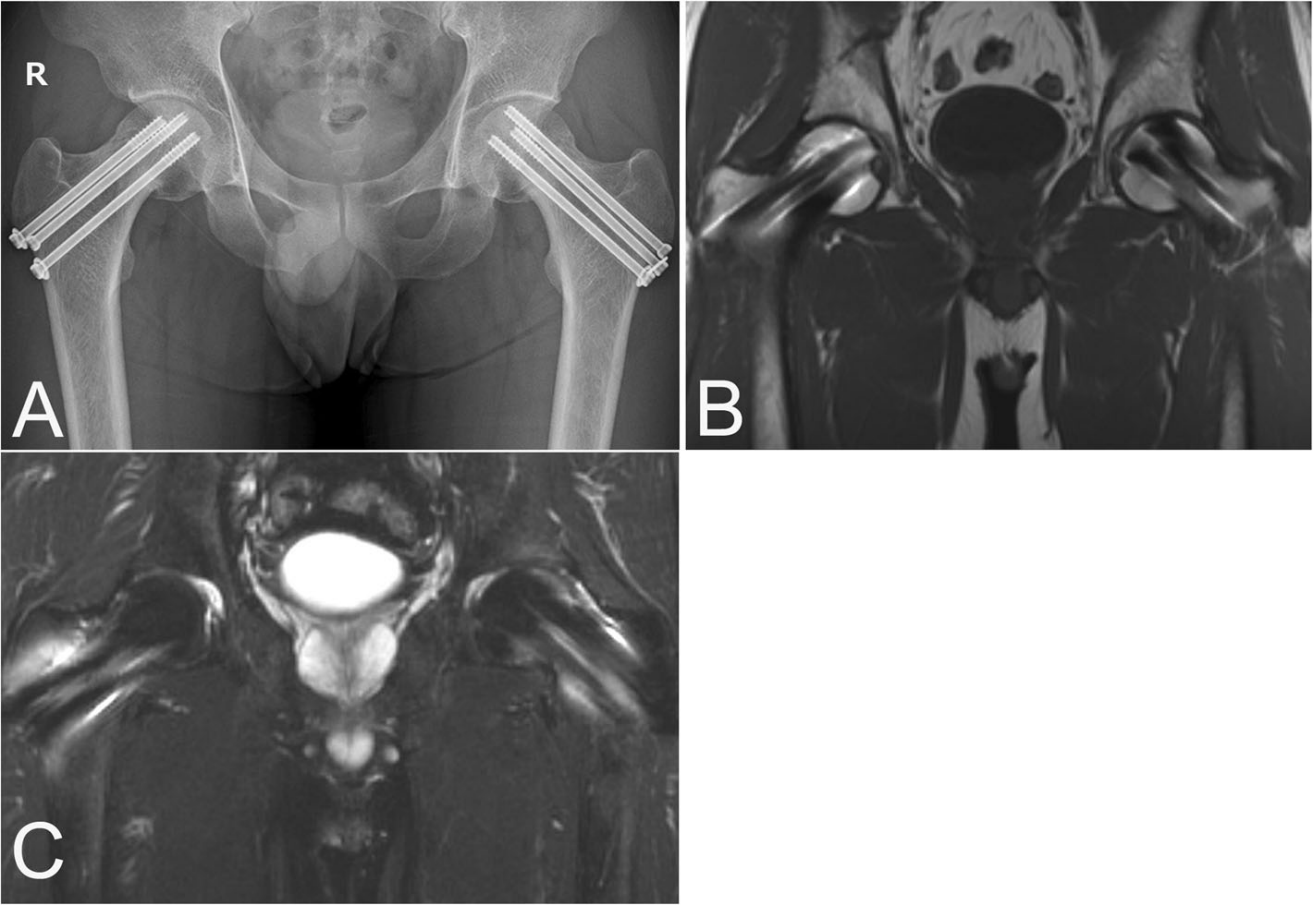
a Bone union of the bilateral femoral neck was confirmed by radiography 8 months after the surgery. No abnormal findings were observed on either coronal T1 (TR/TE = 430/14) (b) or T2 fat-saturated (TR/TE = 4000/84) (c) magnetic resonance images. The bone marrow edema in the bilateral femur and acetabulum disappeared
